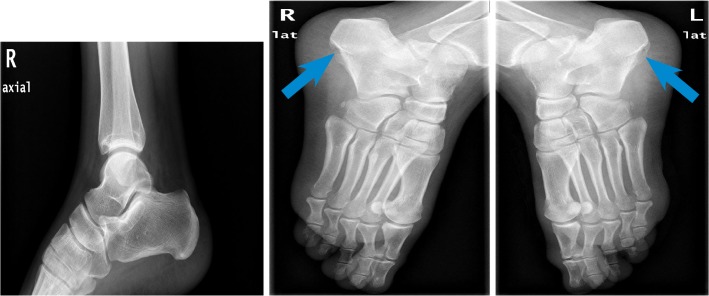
X-rays of the feet during hospital admission showing enthesitis (blue arrows) on both calcanei (film B and C). In contrast, 3 months before she referred to our clinic there is no sign of enthesis involvement (film A).
